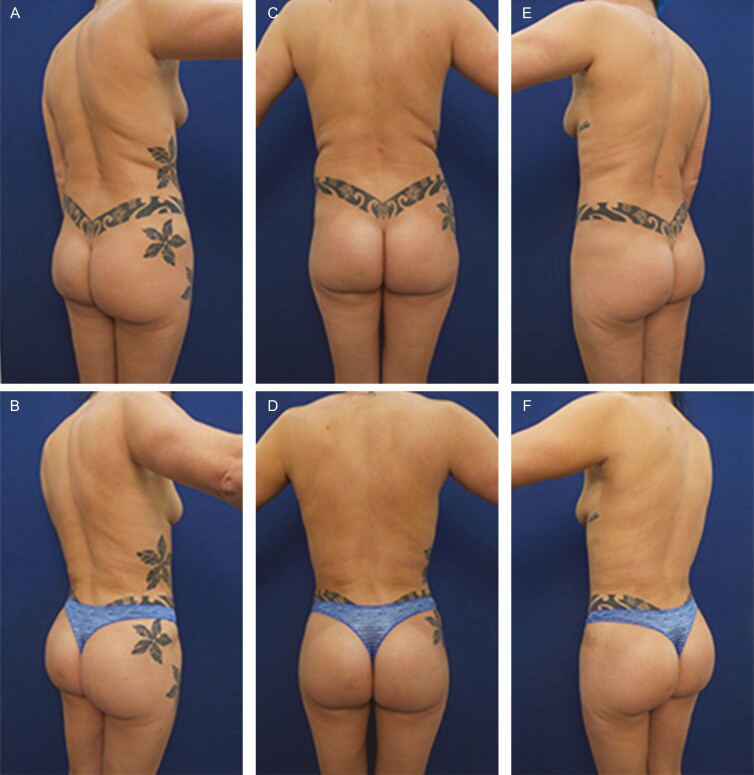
Elimination of back roll following ultrasound-assisted liposuction and helium-activated radiofrequency treatment. This 30-year-old female patient presented with 2 back rolls due to excessive fat and skin redundancy. Successful treatment required liposuction with infiltration and aspiration of 300 cc tumescent solution per side as well as skin tightening using subdermal coagulation. Photographs were taken before and 3 months after surgery. Preoperative and 3 months postoperative (A, B) right lateral view, (C, D) posterior view, and (E, F) left lateral view.
